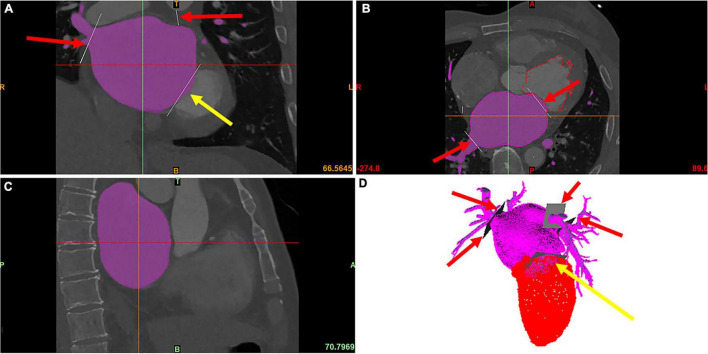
The modeling of LA and LAA. The red arrows point to PVs, and the yellow arrows point to the MV, which is cut manually. The purple area is the LA segmented using the region growing method. (A) The coronal image of cardiac CT. (B) The axial image of cardiac CT. (C) The sagittal image of cardiac CT. (D) The 3D model of LA and LV reconstructed from the cardiac CT.
